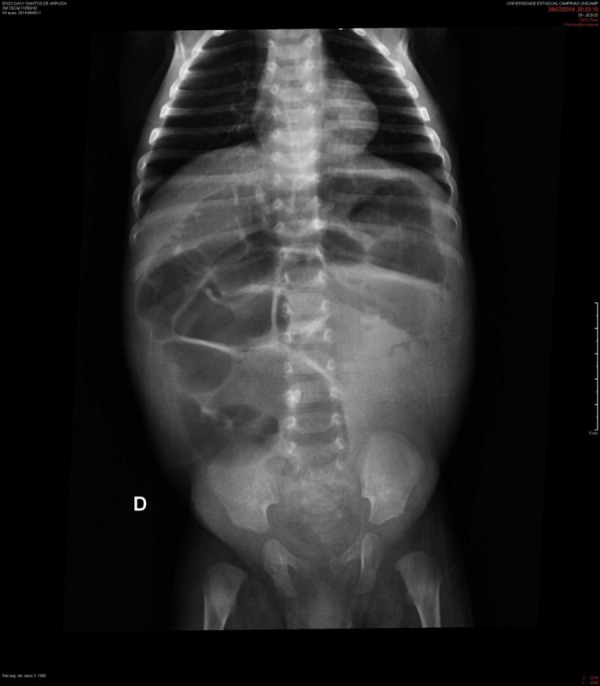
Simple abdominal radiography of a 2-month-old patient with total colon aganglionosis. Preoperative image shows dilated loops of the small bowel and colon, and low volume of air in the colon region.
